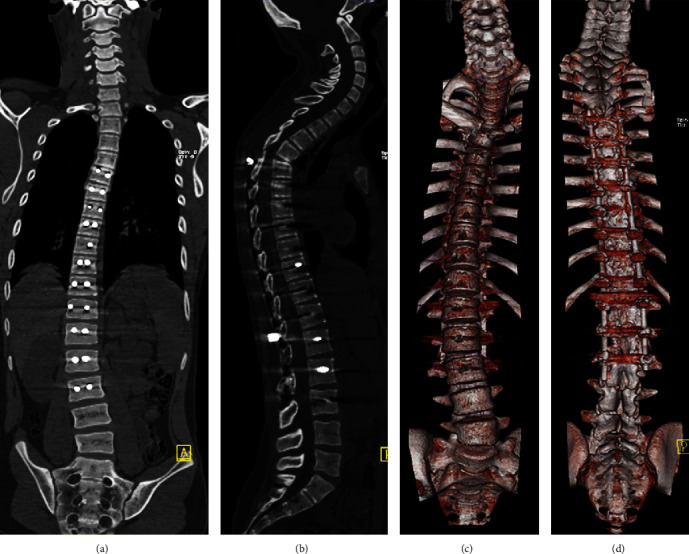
2D and 3D reconstructions of the spine in the bone algorithm show alignment and implant positions after thoracic and lumbar spine surgery. (a, b) Coronal and sagittal views. (c, d) Anterior and posterior aspects of 3D CT reconstructions.
